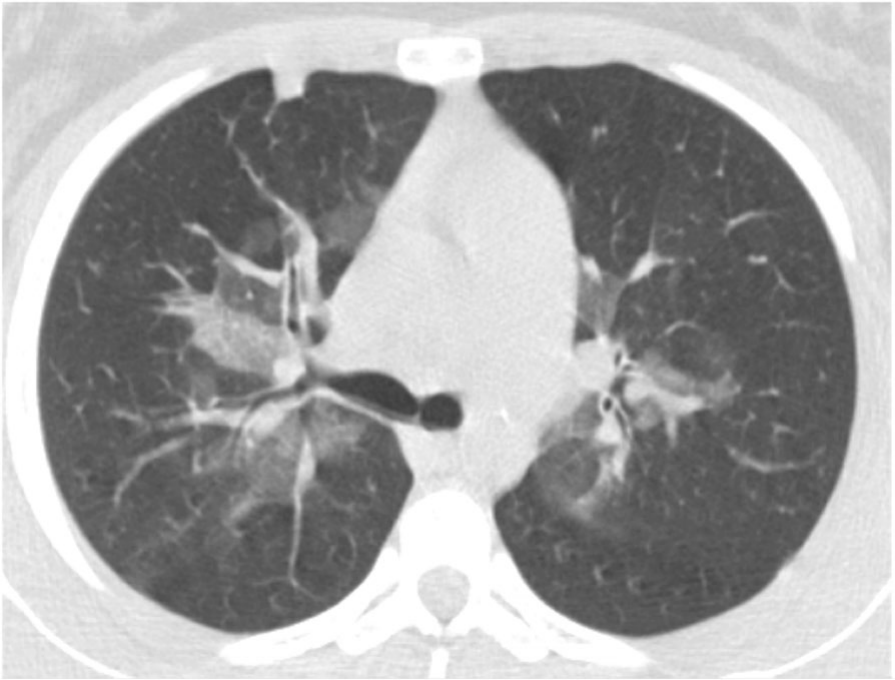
Chest CT (axial view) confirmsing the central ground glass. A small consolidation is evident anteriorly on the right
